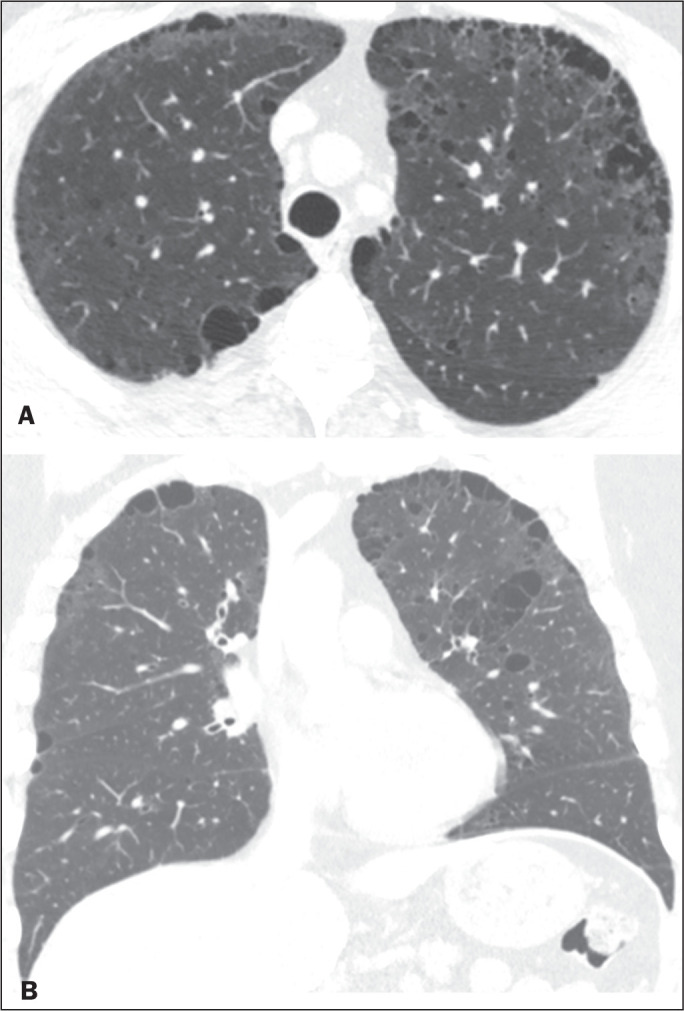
Chest CT of a 45-year-old man with NF1 who was a nonsmoker. Axial and coronal
